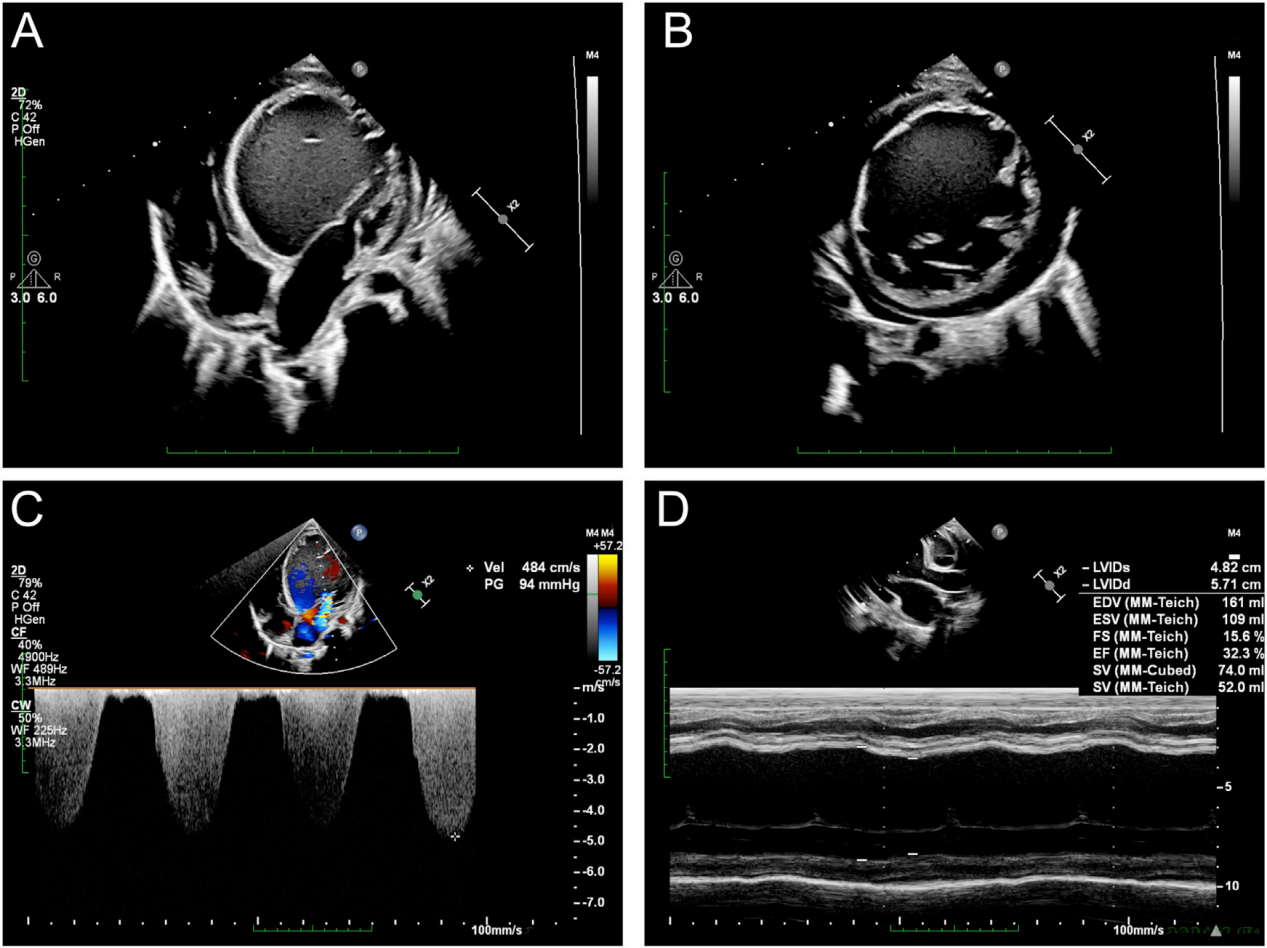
Echocardiographic assessments of the proband: (A) cardiac ultrasound demonstrates significantly enlarged left ventricle; (B) short-axis image reveals a hypertrabecularization in the left ventricle; (C) severe regurgitation observed at the mitral valve; (D) severe heart failure recorded by M-mode ultrasound.
